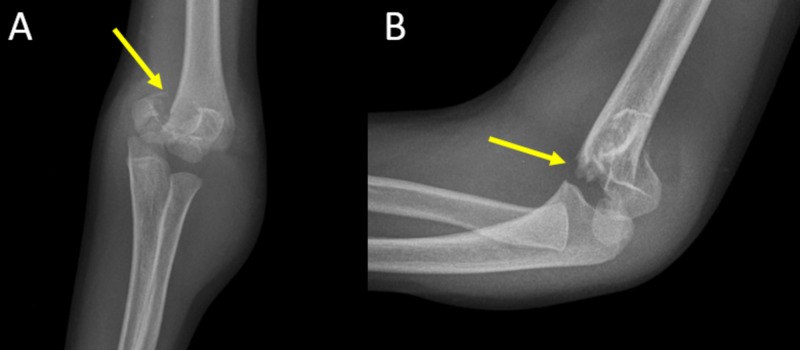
Anteroposterior (A) and lateral (B) x-ray view of the elbow of a five-year-old boy with a displaced extension type supracondylar fracture Arrows indicate the location of the fracture.
